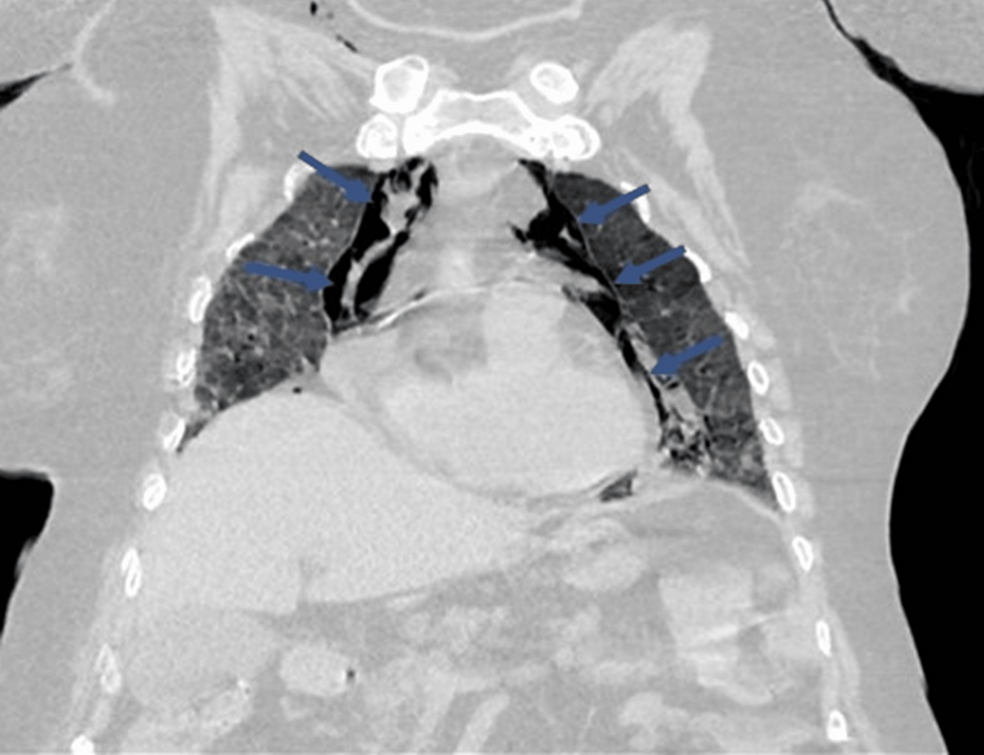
Computed tomography of the chest (coronal section) The image is revealing a large pneumomediastinum and cervical emphysema.
